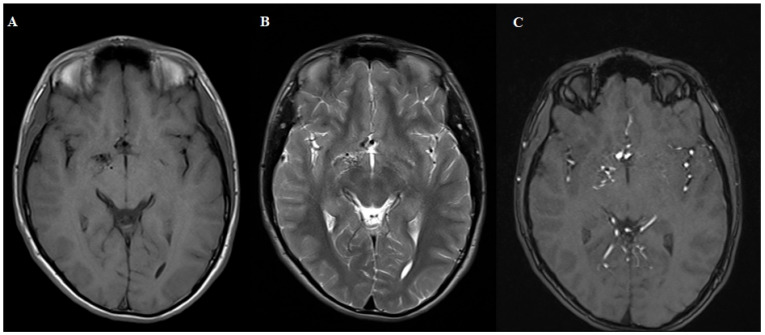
MRI T1 (A), T2 (B) and 3D TOF (C). Sequences demonstrating normal brain parenchyma with a tangle of small blood vessels without a visible draining vein. The patient was referred for digital subtraction angiography.
